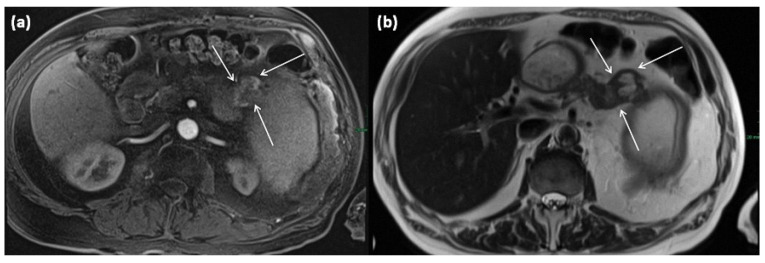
MRI of a 60-year-old patient with a partly cystic and partly solid mass of the pancreatic tail. Arterial T1w after gadolinium-based contrast administration (a) typical peripheral hyperarterilization (arrows) can be seen. In T2w, solid as well as cystic parts are present (b).
